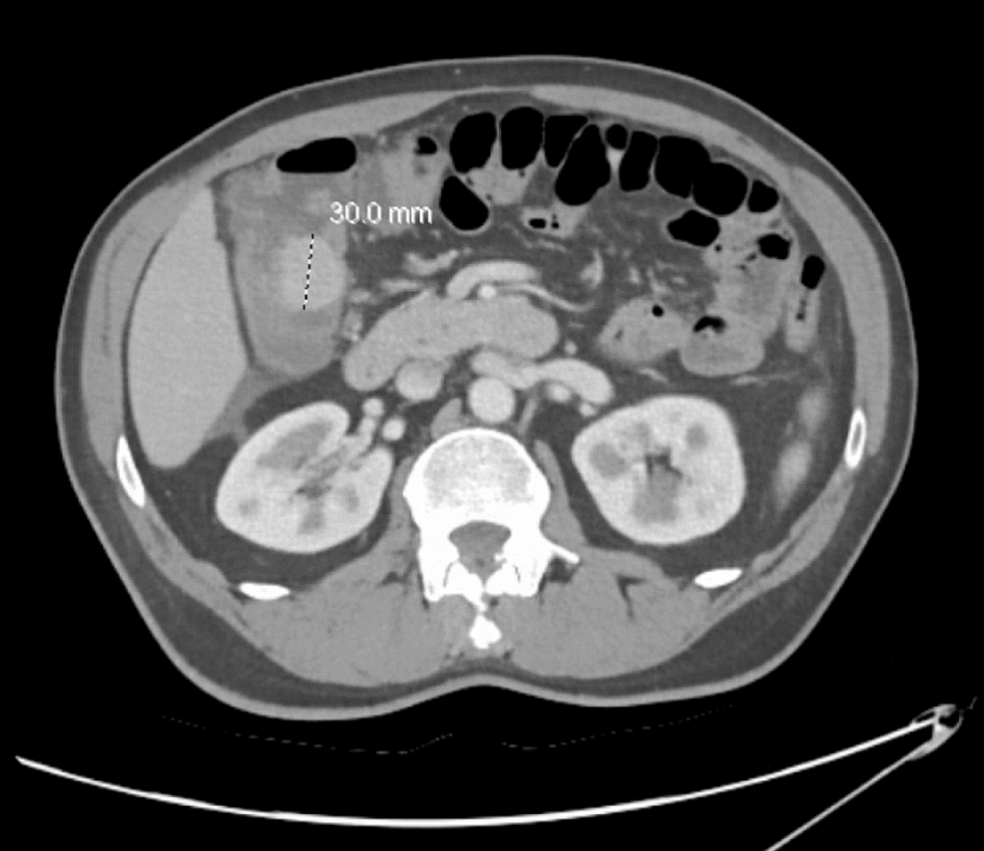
CT of the abdomen and pelvis with intravenous contrast, axial view.
